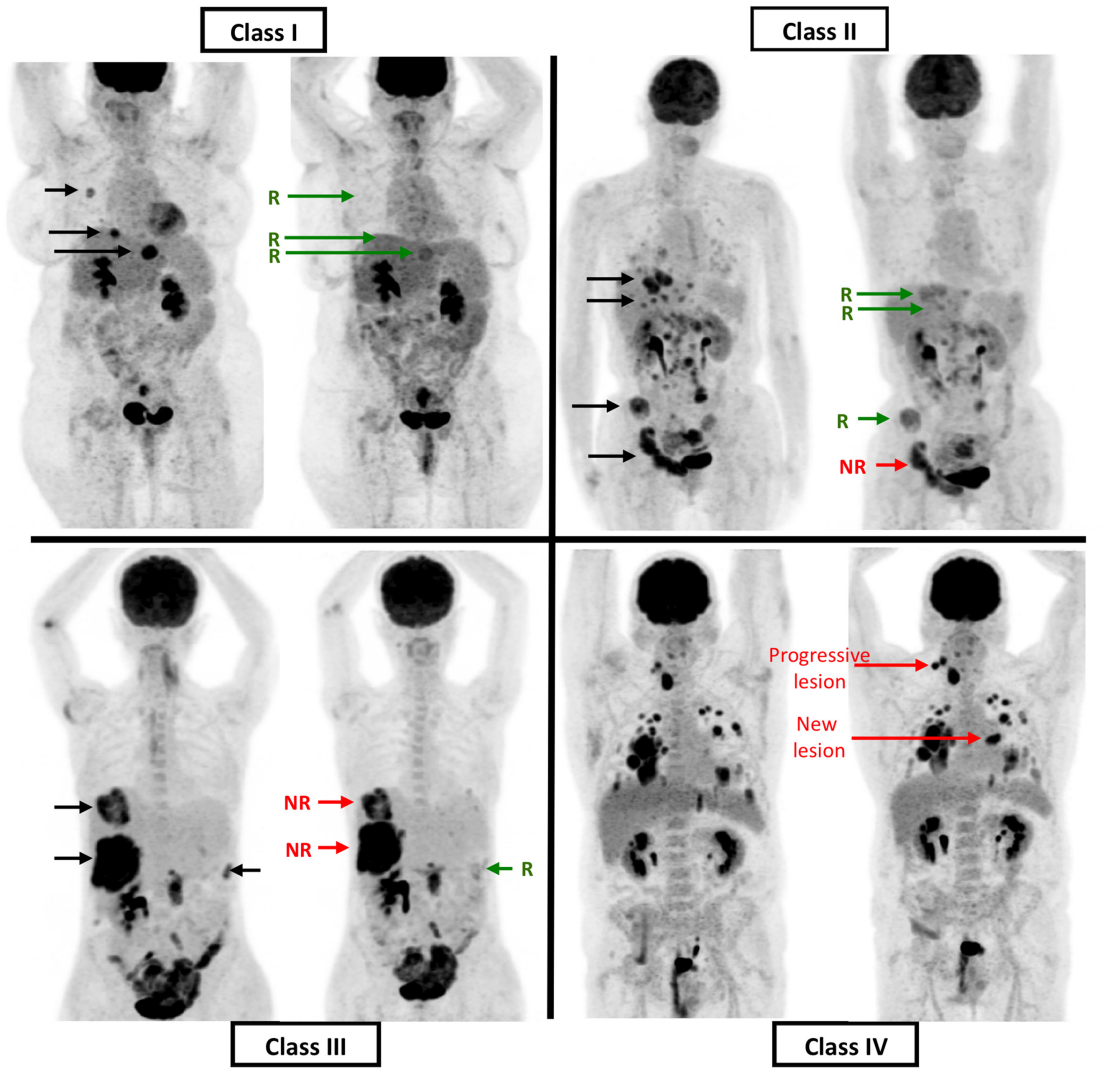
Classes of metabolic responses. Class 1: no metabolic unresponsive lesion; Class 2: minority of unresponsive lesion among whole body target tumour load; Class 3: majority of whole body target tumour load does not respond; Class 4: all target lesions are non-responding, or, presence of progressive lesions [progression defined as >25% increase of FDG uptake on second PET, or appearance of a new lesion].
